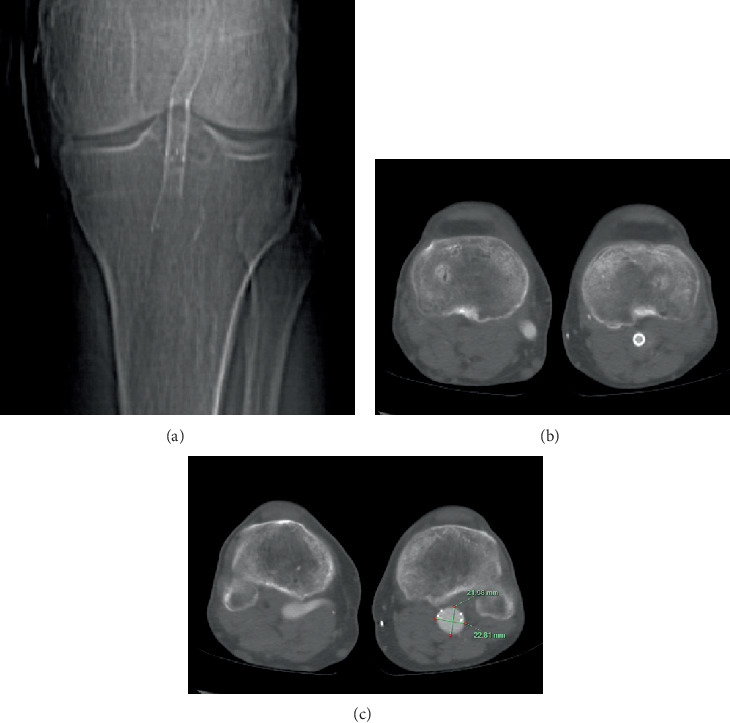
Computed tomography angiogram. Scout image demonstrating (a) fractured stent, (b) with the superficial artery stent patent just above the level of the popliteal artery aneurysm and (c) subsequent aneurysmal dilation of approximately 23 × 22 mm.
